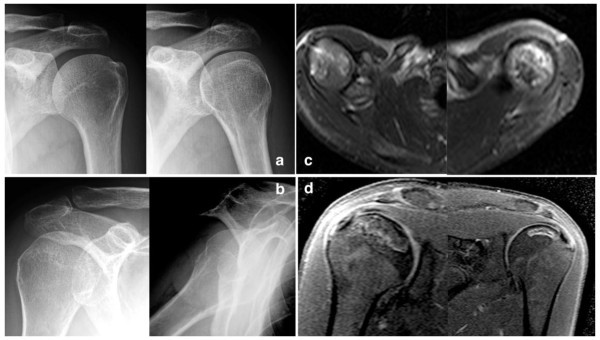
A 28-year-old patient with a history of acute lymphoblastic leukemia and hip osteonecrosis presented with painful shoulders. The plain x-rays (a, b) were negative. The axial fat suppressed T2-w TSE MR images (c) showed a small ON lesion in both humerus head. Because of the severity symptoms it was decided to undergo to the surgical treatment with core decompression. For preoperative planning reasons he underwent to a typical MRI (T1-W STIR) in order to have a more accurate evaluations of the lesions size (d).
